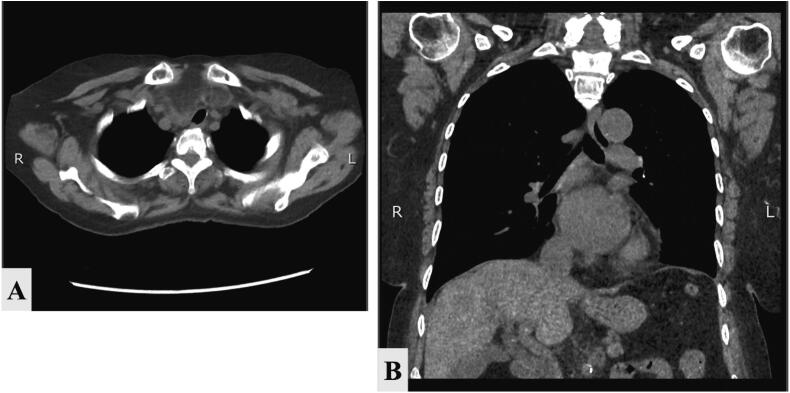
A: Preoperative CT scan showing minimal retrosternal extension of the multinodular goiter. A: Axial view. B: coronal view.
